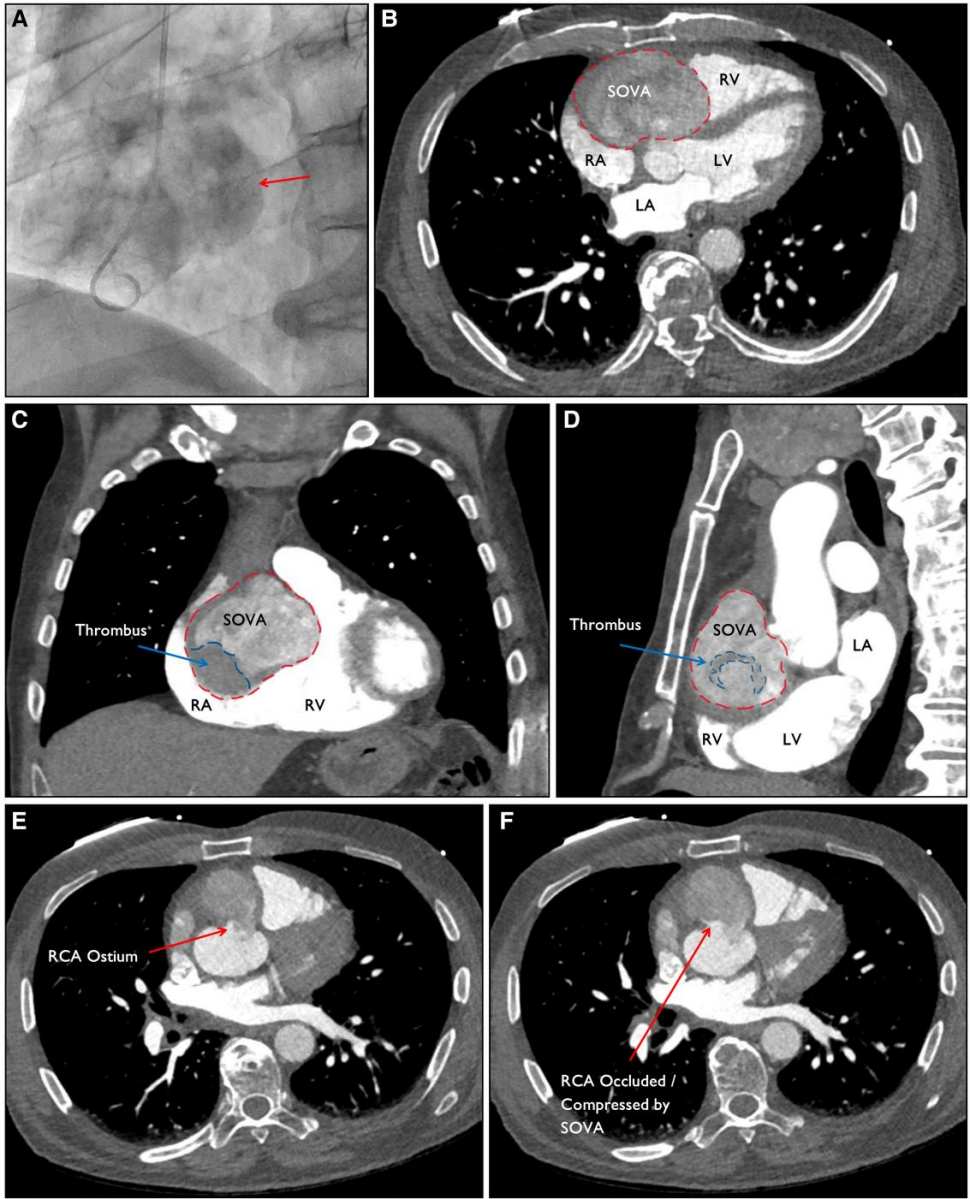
(*A*) Coronary angiogram demonstrated pooling of contrast adjacent to the right coronary cusp (arrow), indicating a dissection/aneurysm. (*B–D*) Computed tomogram aortogram showed a 50 × 67 × 76 mm large aneurysmal dilatation from the right aortic sinus, representing a right sinus of Valsalva aneurysm (dotted red, outer line). Heterogenous contrast mixing within the structure represents probable thrombi (dotted blue, inner line). (*E* and *F*) Transverse slices of the CT chest demonstrating the presence of the right coronary artery ostium, with its eventual occlusion due to probable thrombus or compression by the aneurysm (arrows).
